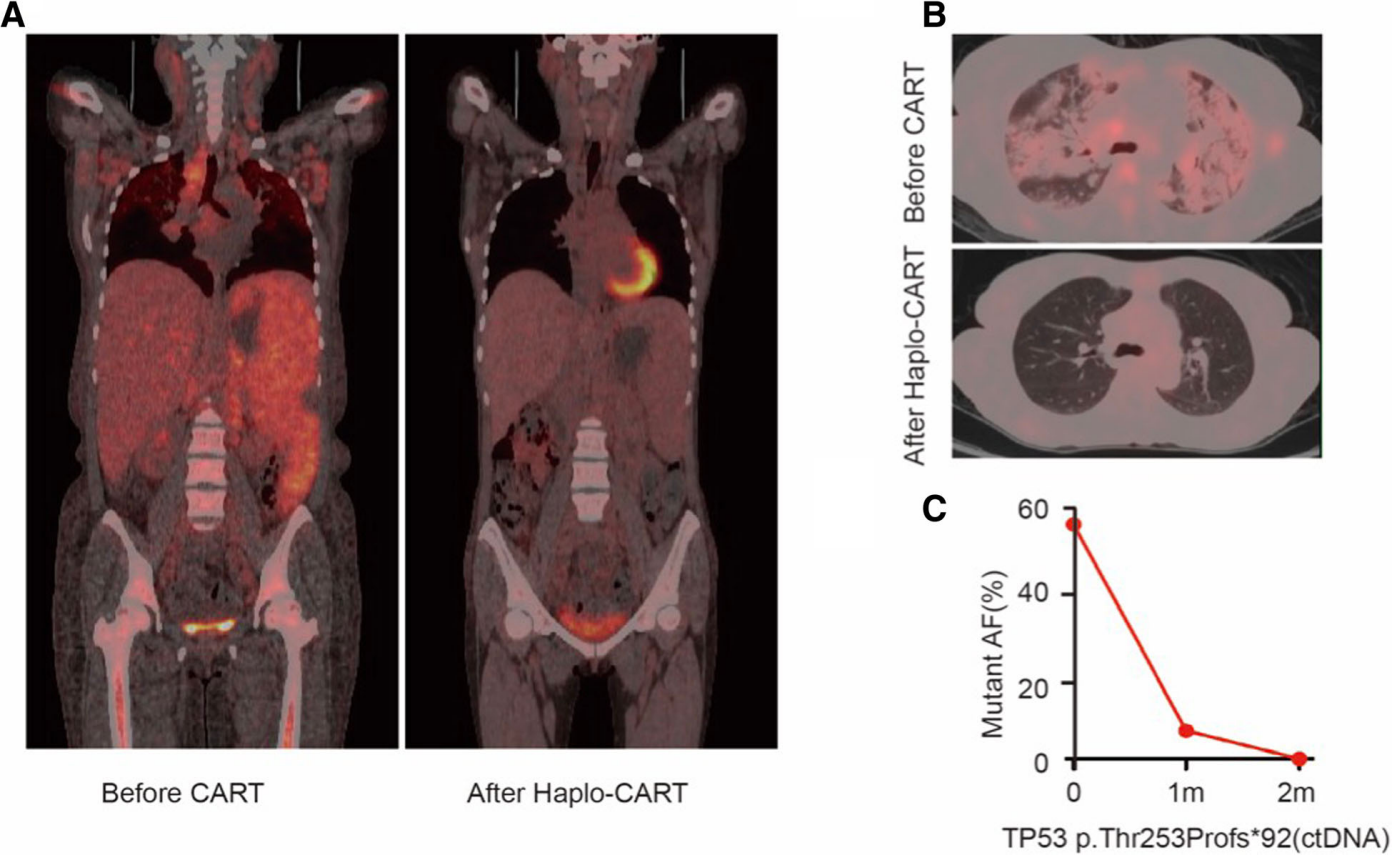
PET/CT and liquid biopsy monitoring the efficacy of haplo-CAR T cell therapy. a Whole body PET/CT image before CART therapy and 45 days after haplo-CAR T cell therapy. b Lung slice of tumor infiltration before CAR T cell therapy and 45 days after haplo-CAR T cell therapy. c The mutant percent of TP53 gene p.Thr253Profs*92 in circulating tumor DNA by liquid biopsy before CAR T cell therapy, in 1 and 2 months after haplo-CAR T cell therapy
